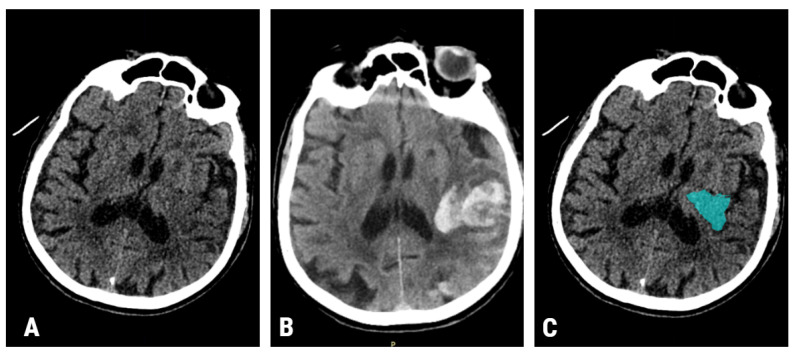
Representative case of a 72-year-old patient with acute left insular ischemic stroke and subsequent HT. (A) Baseline non-contrast CT demonstrating subtle hypoattenuation in the left insular region, consistent with early ischemic changes. (B) Follow-up CT at 24 h showing HT within the infarcted territory. (C) Region of interest (ROI) placed on the baseline CT, corresponding to the infarcted area that subsequently developed hemorrhage on follow-up imaging, used for texture analysis.
